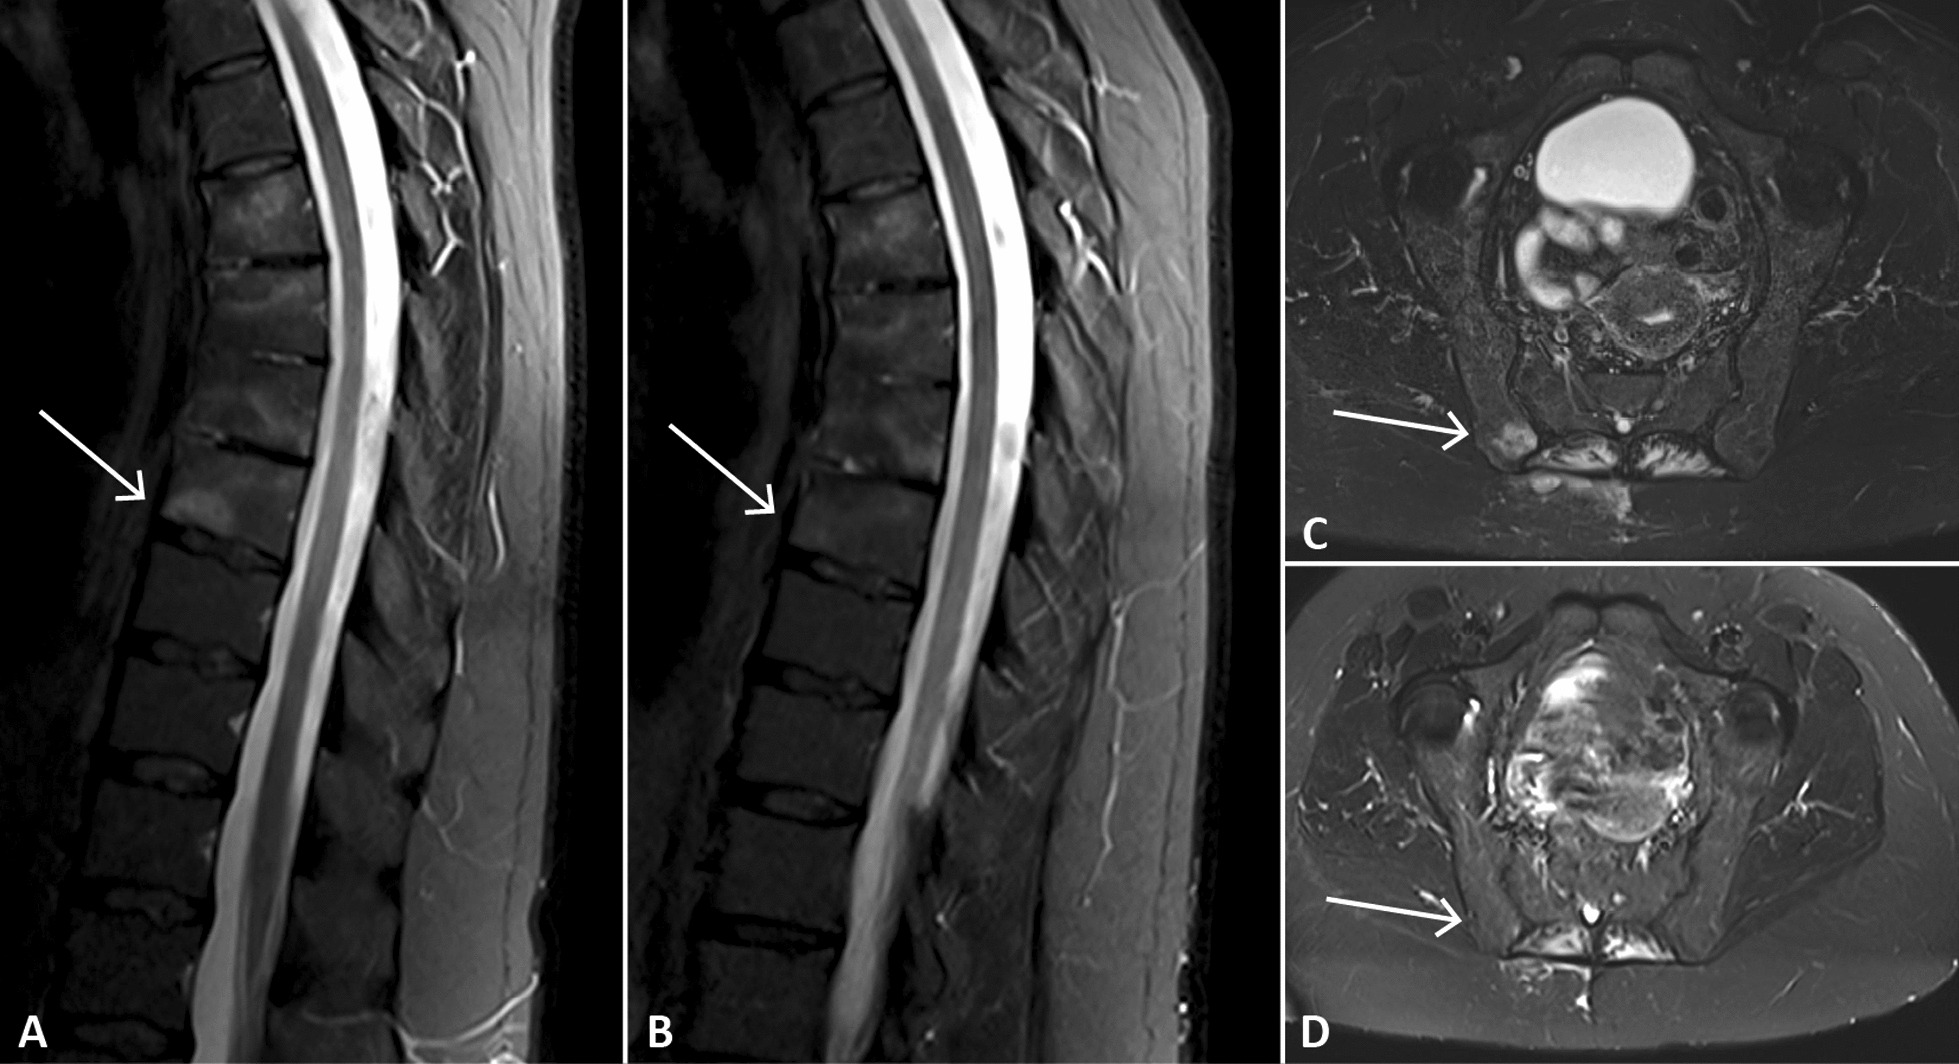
A, B Magnetic resonance imaging (MRI) of the thoracic spine before and 3 months after the onset of treatment with tofacitinib. C, D MRI of the iliac bone before and 3 months after the onset of treatment with tofacitinib. Arrows show the bone marrow oedema
